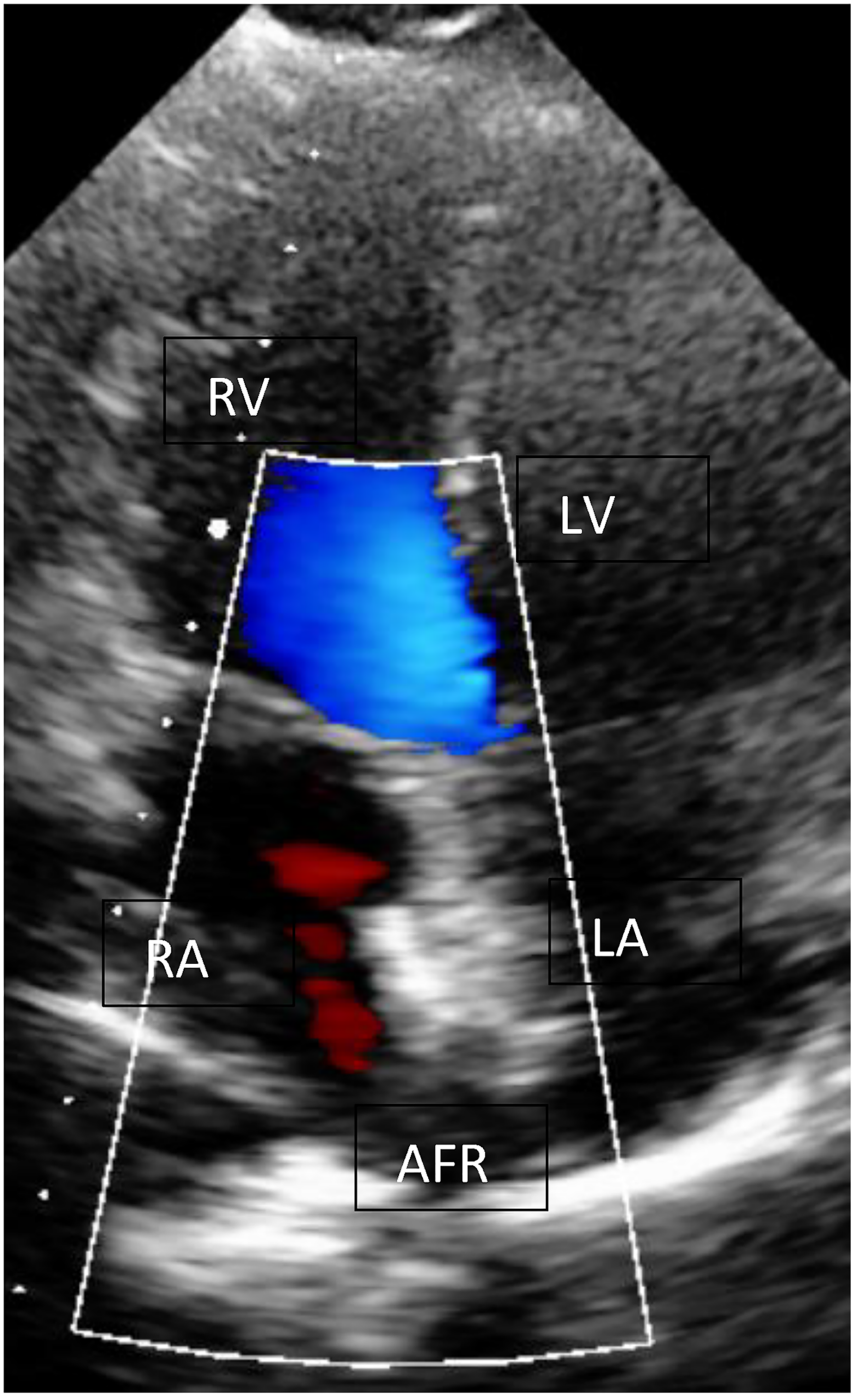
AFR device (Occlutech, Germany) in echocardiography. AFR device (Occlutech, Germany) with L-R shunt at rest; RA, right atrium; LA, left atrium; LV, left ventricle; RV, right ventricle.
